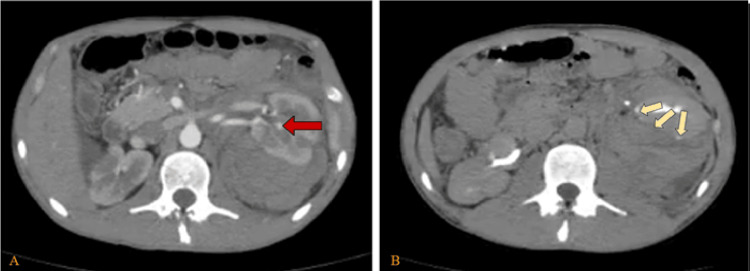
Abdomen and pelvis CT with IV contrast on day 2 (A) Arterial phase showing a huge left perinephric retroperitoneal hematoma with no change in its size with little arterial blush (red arrow). (B) Delayed phase showing a rim of contrast extravasation around the left kidney (yellow arrows) with suspected cortical defect reaching its pelvic seen in the midportion, an hypoenhancing area in the upper pole, suggesting urinary extravasation
